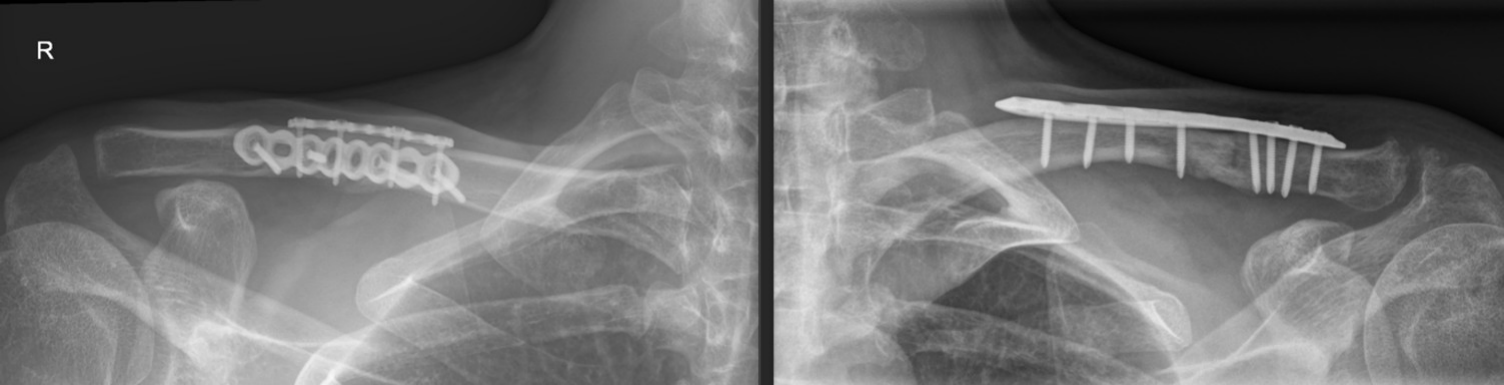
Postoperative radiographs of both techniques. Left: Double plating. Right: Single plating.
